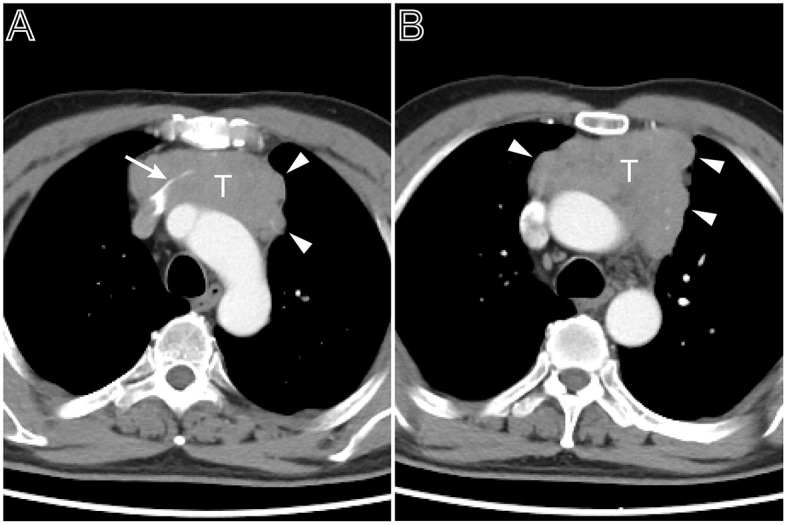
A multi-lobulated heterogeneous thymoma with vascular invasion. Axial contrast-enhanced chest CT image obtained at the level of the aortic arch demonstrated a thymoma in a 54-year-old man complained of bosom frowsty and chest pain (Masaoka–Koga stage III and WHO classification B1). The lesion directly invaded the left brachiocephalic vein (A, white arrow). The tumor showed the heterogeneous attenuation distributions and multi-lobulated contour (A,B, arrow head), the aortic arch was pushed backward.
